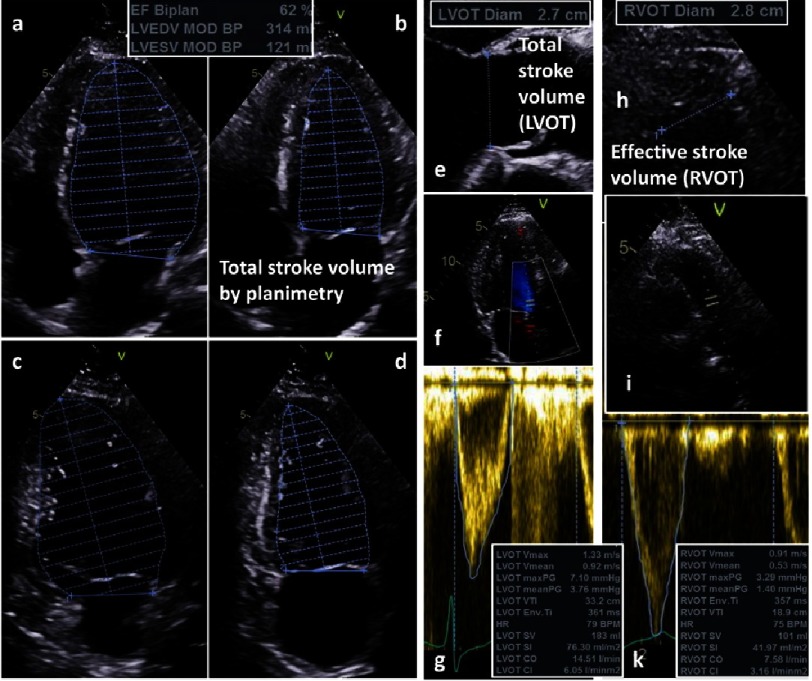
Conventional assessment of total stroke volume (SV_t_) and effective stroke volume (SV_e_) by 2D-echocardiography; determination of SV_t_ by LV planimetry using the modified Simpsons’ rule (4-chamber view: a - diastole; b - systole; 2-Chamber view: c - diastole, d -systole) and by Doppler echocardiography (e - LVOT diameter; f - position of the sample volume; g - pulsed-wave Doppler spectrum and VTI of LVOT flow velocities); determination of SV_e_ by Doppler echocardiography (h - RVOT diameter; i - position of the sample volume; k - pulsed-wave Doppler spectrum and VTI of RVOT flow velocities).
